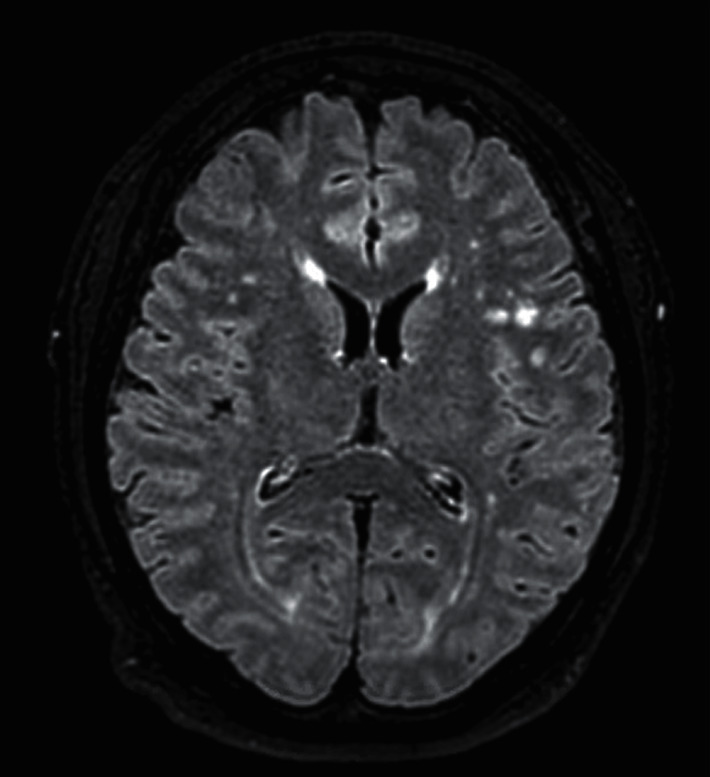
MRI brain axial FLAIR. Multifocal supratentorial ovoid and nonspecific T2 hyperintensities. Red arrow points to an ovoid T2 hyperintensity.
